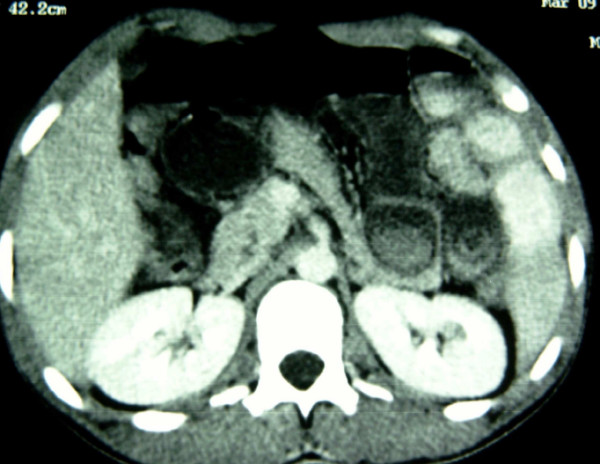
CT of the abdomen demonstrating classic target sign in the the left upper quadrant, pathognomonic for ileoileal intussusception.
